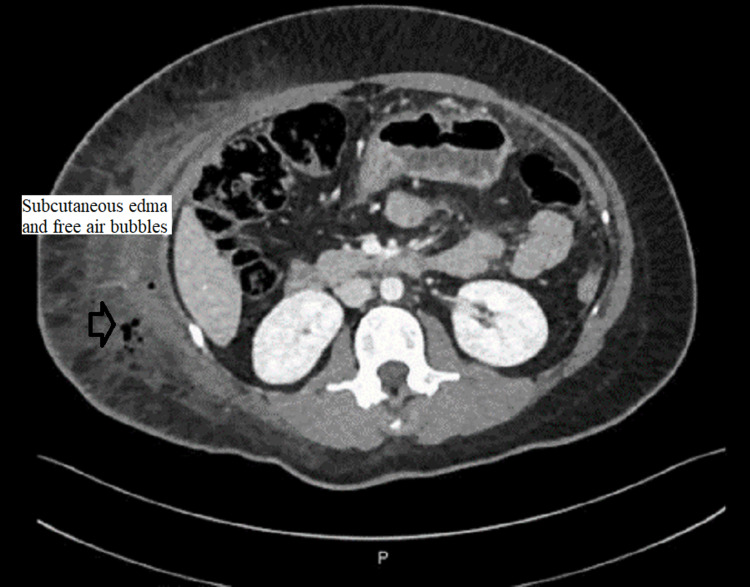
CT abdomen showing extensive edema, fat stranding, and inflammatory changes seen at the right lateral abdominal wall with free air in the subcutaneous soft tissue and muscular planes
